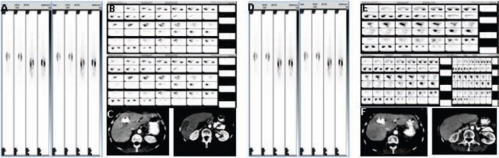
Best objective response in a patient affected by liver metastases from non-functioning endocrine pancreatic carcinoma, treated with 90Y-DOTATOC. At the enrolment the patient had progressed elsewhere after trans-arterial chemo-embolisation, performed six months before (a, b and c: basal whole-body scan, anterior and posterior view, SPECT sections and CT sections, respectively; d, e and f: whole-body scan, anterior and posterior view SPECT sections and CT sections after therapy).
